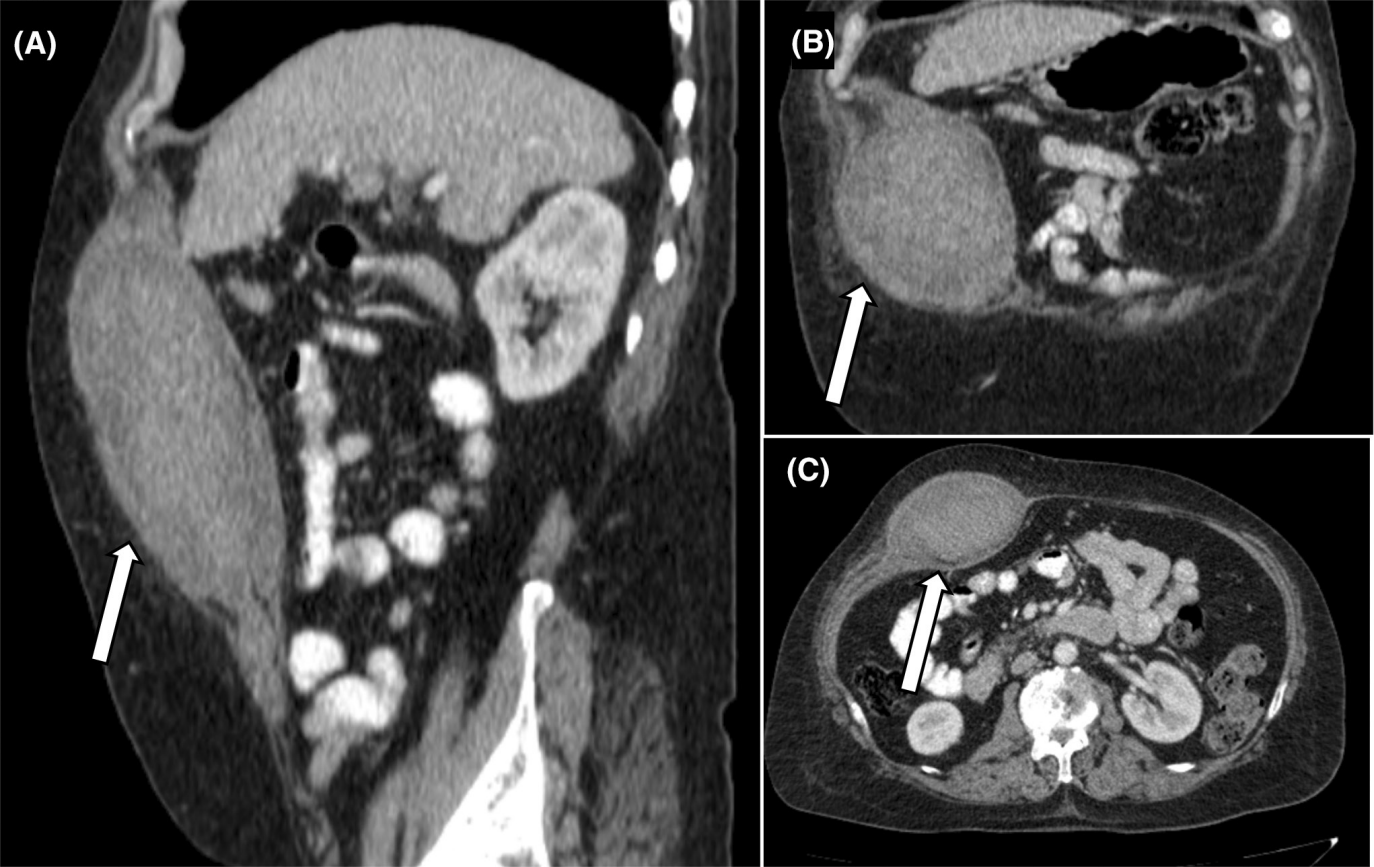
Sagittal reformatted (A), coronal reformatted (B), and axial (C) images of CT abdomen showing heterogeneously hyperdense lesion (white arrows) in right rectus abdominis muscle and nodular shrunken liver in (A) and (B).
